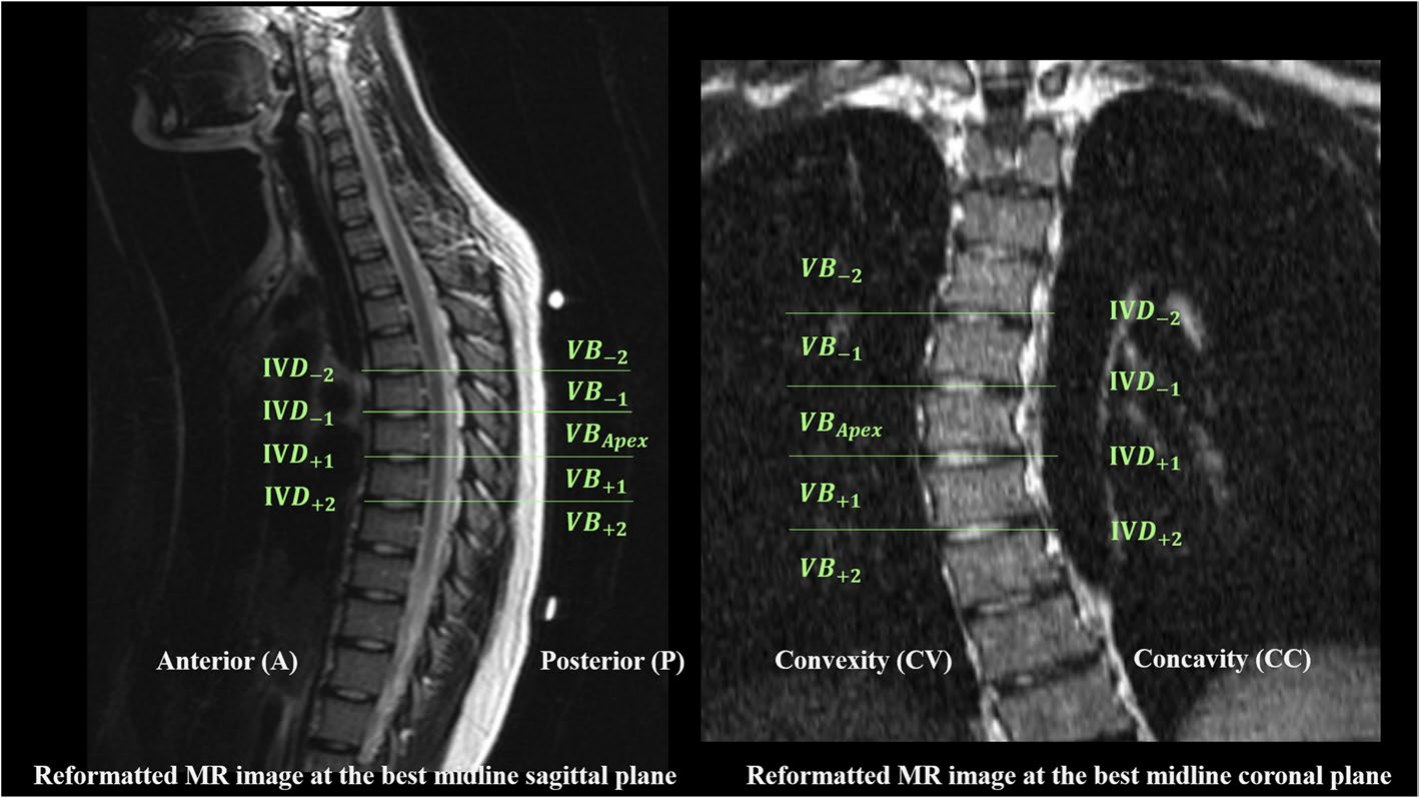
A T2-weighted MR reformatted at the best midline coronal and sagittal planes of a 15-year-old female AIS patients. The nomination of vertebrae (VB) and intervertebral discs (IVD) are shown
